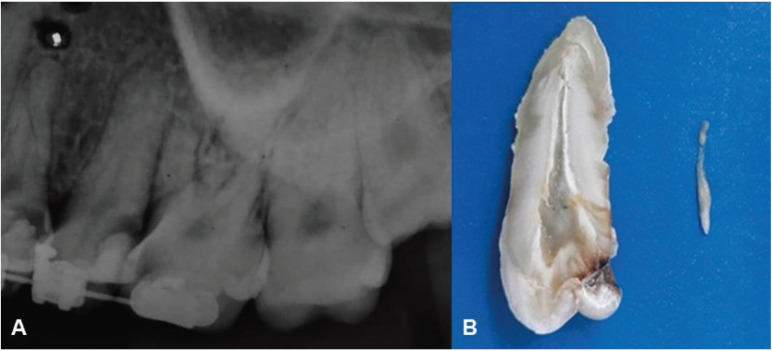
A. Periapical radiograph showing an extensive deep carious lesion on the oclusal and distal surfaces of the upper left second premolar (tooth #25). B. Upper left second premolar extracted and cleaved in the buccal-lingual orientation, showing the deep carious lesion and the pulp tissue removed.
